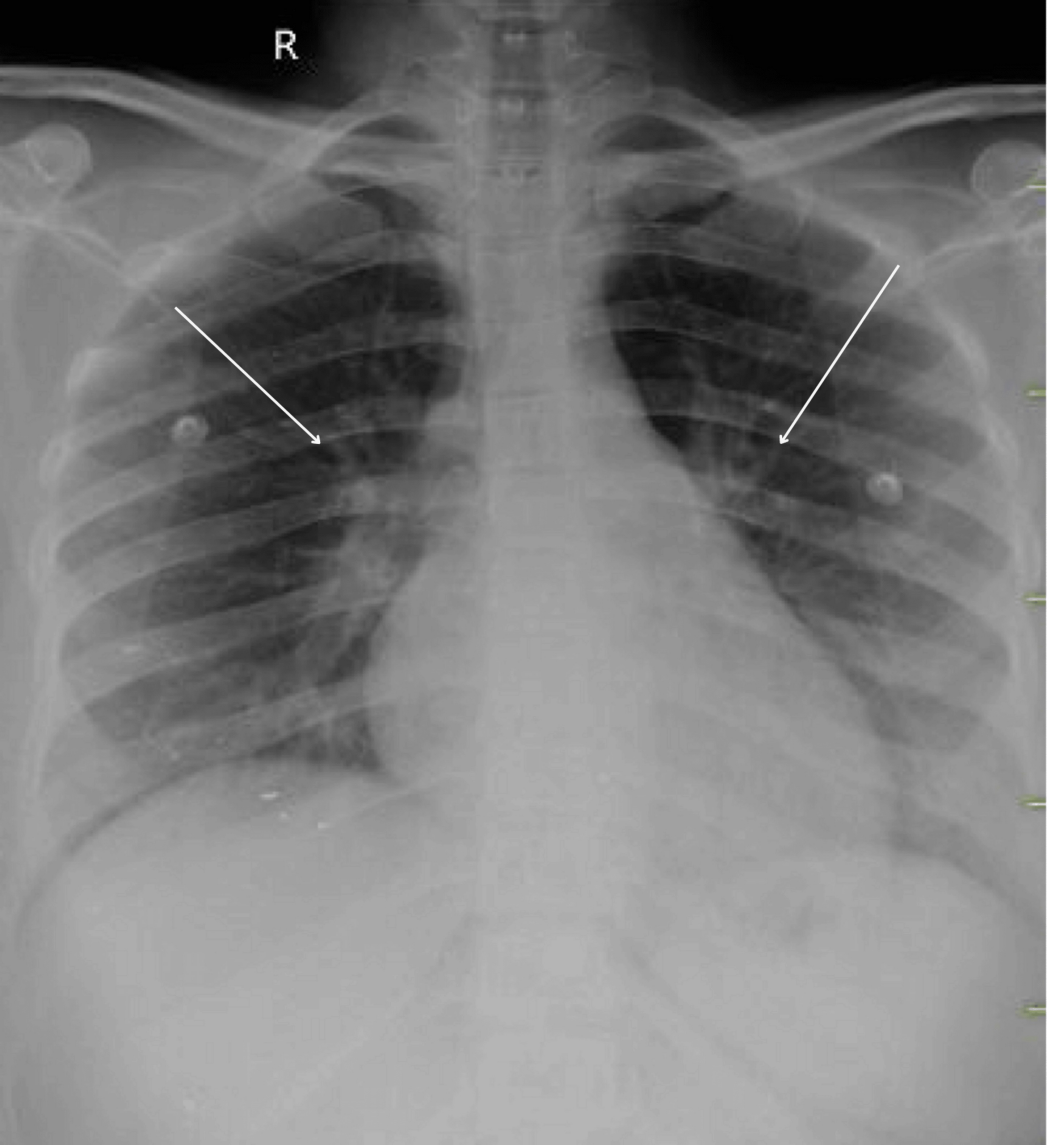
Chest radiograph of the patient showing decrease in the pulmonary edema (white arrows)
